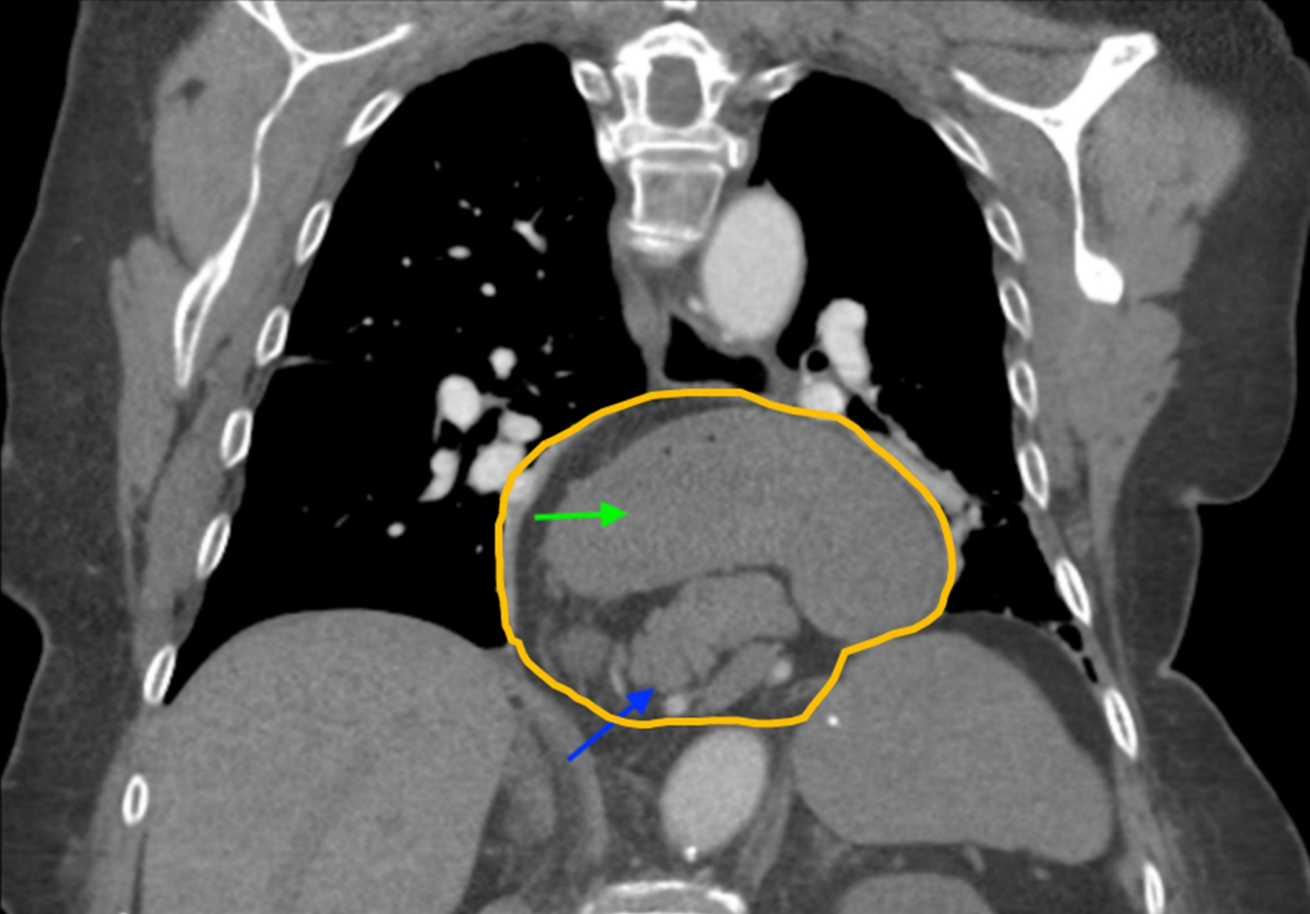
Coronal CT angiogram of the chest featuring a massive type IV hiatal hernia with intra-abdominal contents A massive type IV hiatal hernia (orange outline) containing a large portion of the pancreas (blue arrow) and stomach (green arrow) is shown entering the thorax and obscuring the view of critical vasculature.
